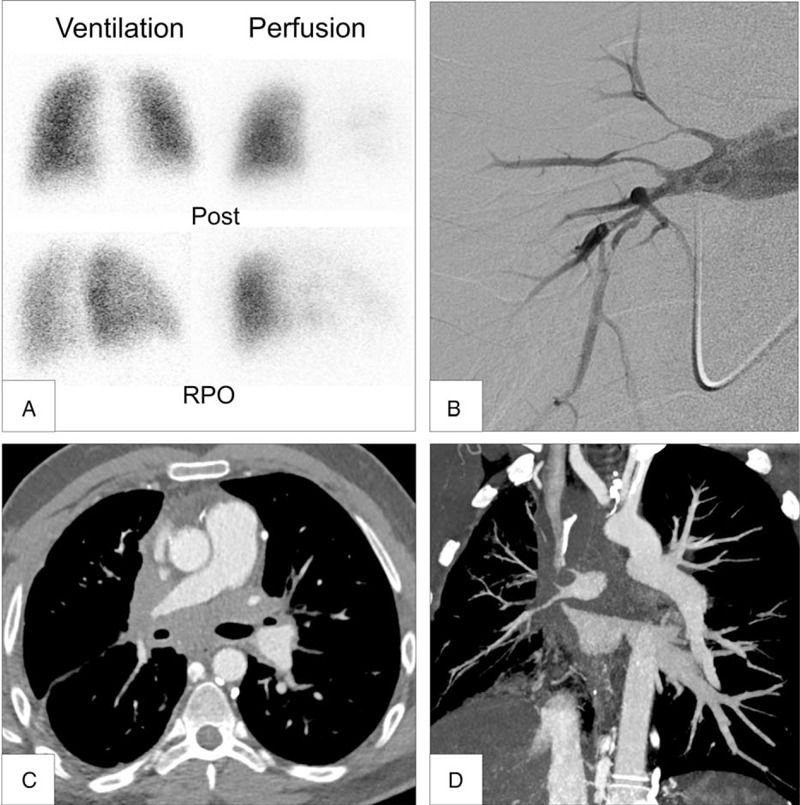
V/Q lung scan, contrast-enhanced high-resolution computed tomography and pulmonary angiography in a patient with pulmonary hypertension complicating fibrosing mediastinitis masquerading as chronic thromboembolic pulmonary hypertension. Panel A: V/Q lung scan showing multiple segmental perfusion defects in contrast with normal ventilation. Panels B: selective right pulmonary angiogram showing filiform right pulmonary arteries. Panels C and D: cross-sectional and coronal views of contrast-enhanced high-resolution computed tomography showing extrinsic pulmonary arterial compression by fibrosing mediastinitis. Post = posterior view on V/Q lung scan; RPO = right posterior oblic view on V/Q lung scan.
